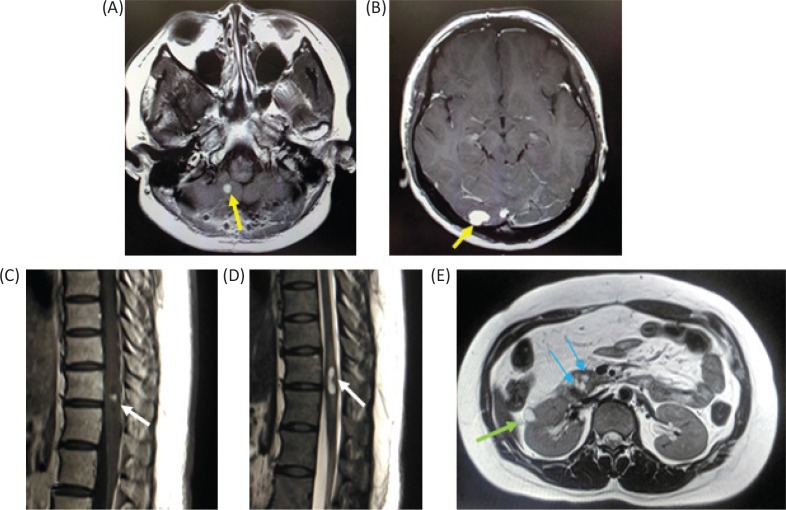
Magnetic resonance imaging of the patient. (A and B) Gadolinium (Gd)-enhanced T1-weighted image of cerebellum when the patient was 26 years old. The patient had multiple cerebellar hemangioblastomas. (C) Gd-enhanced T1-weighted image of the lower thoracic cord. A small hemangioblatoma with a syrinx is shown. (D) T2-weighted image of the lower thoracic cord. (E) T1-weighted image of the abdomen. A renal cyst and multiple pancreatic cysts are shown.
